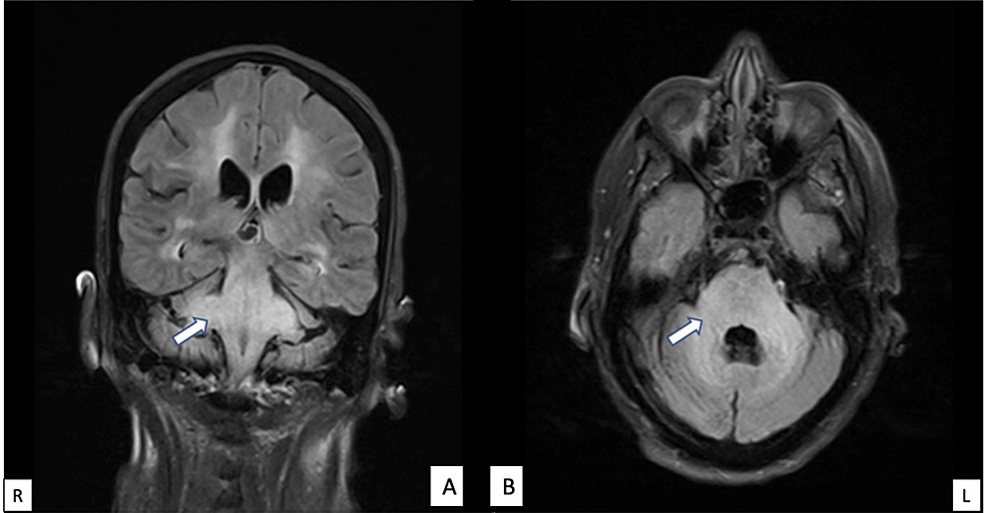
Extensive FLAIR hyperintensities are demonstrated within the subcortical and periventricular white matter, brainstem, middle cerebellar peduncles and cerebellar hemispheres, with moderate improvement of T2/FLAIR hyperintense signal within the brainstem: (A) coronal view, (B) axial view. FLAIR: fluid-attenuated inverted recovery.
